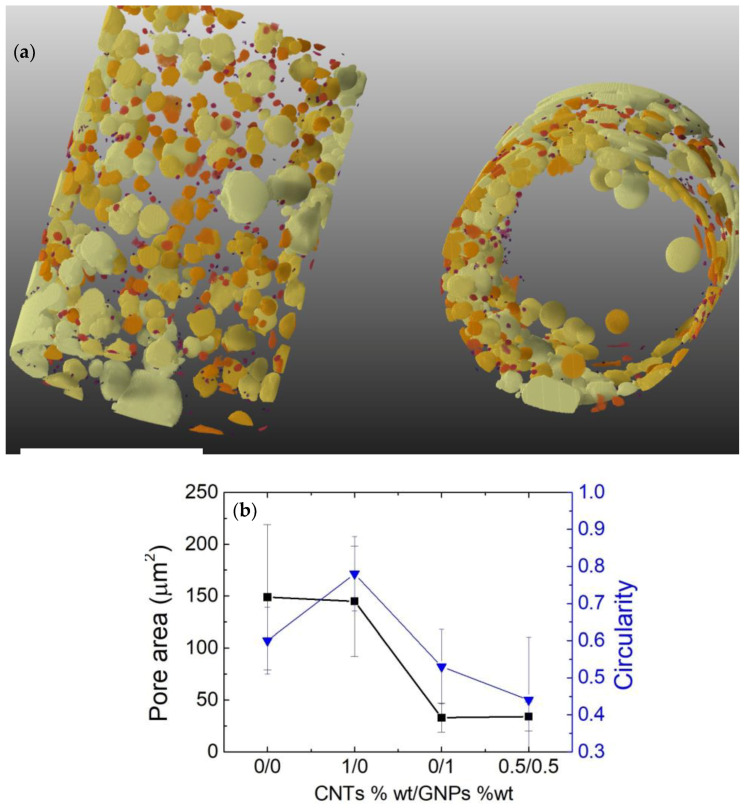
(a) Snapshots of μ-CT analysis showing the volume distribution of the bubbles around the hollow middle cylinder. The scale bars indicate 10 mm. (b) Pore area and circularity data of the pores as a function of the CNTs/GNPs weight ratio.
